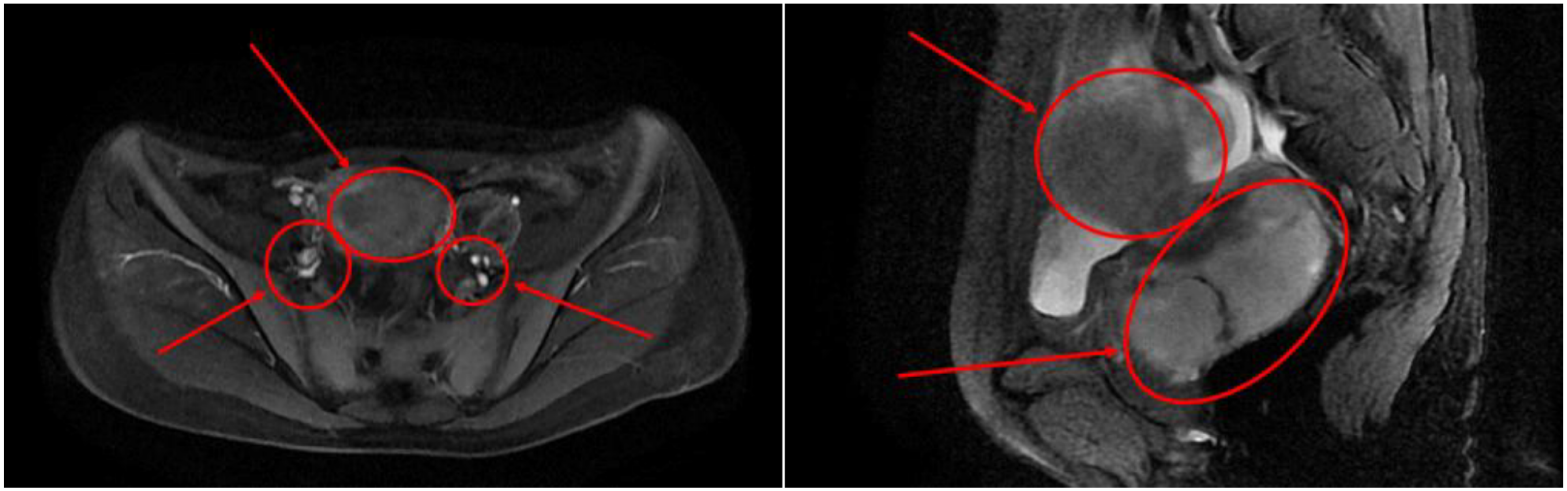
Cross-sectional pelvic MRI and sagittal pelvic MRI findings.
